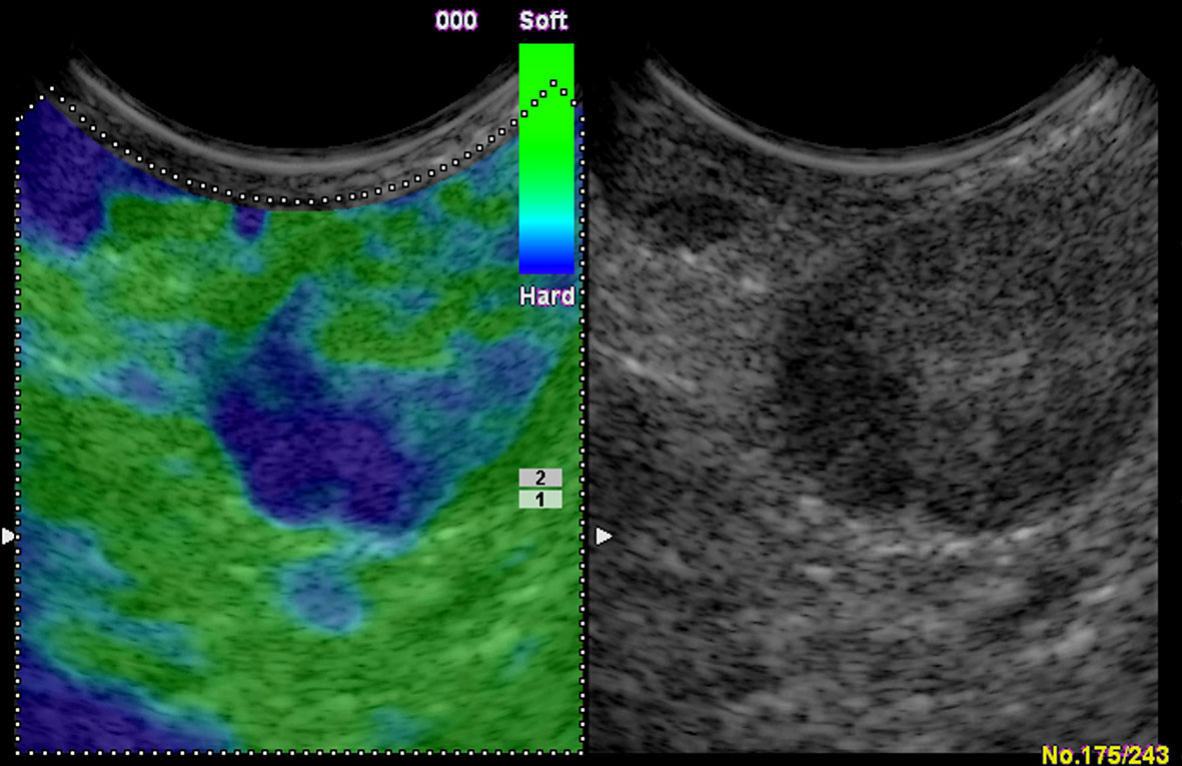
Colorectal carcinoma with presacral circumscribed lymph node metastasis in endoscopic ultrasound. The strain elastography reveals a typically harder (blue) area in the lymph node.
